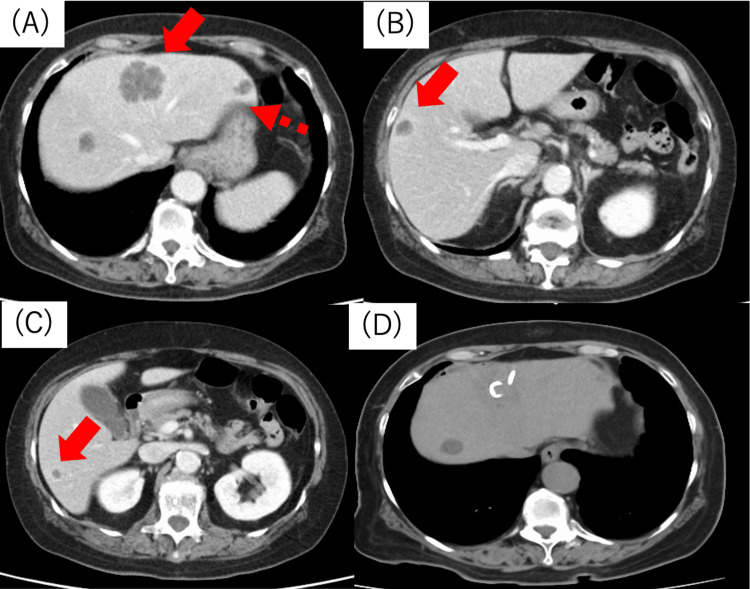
Image of a CT scan taken upon admission at the referring hospital. Contrast-enhanced CT imaging showing (A) a multifocal abscess in hepatic segment S4 (40 mm) (arrow) and an abscess in S3 (point arrow). Additional abscesses were observed in (B) S5 (arrow) and (C) S6 (arrow). (D) Percutaneous transhepatic drainage was performed to treat the multifocal abscess in hepatic S4 CT: computed tomography
